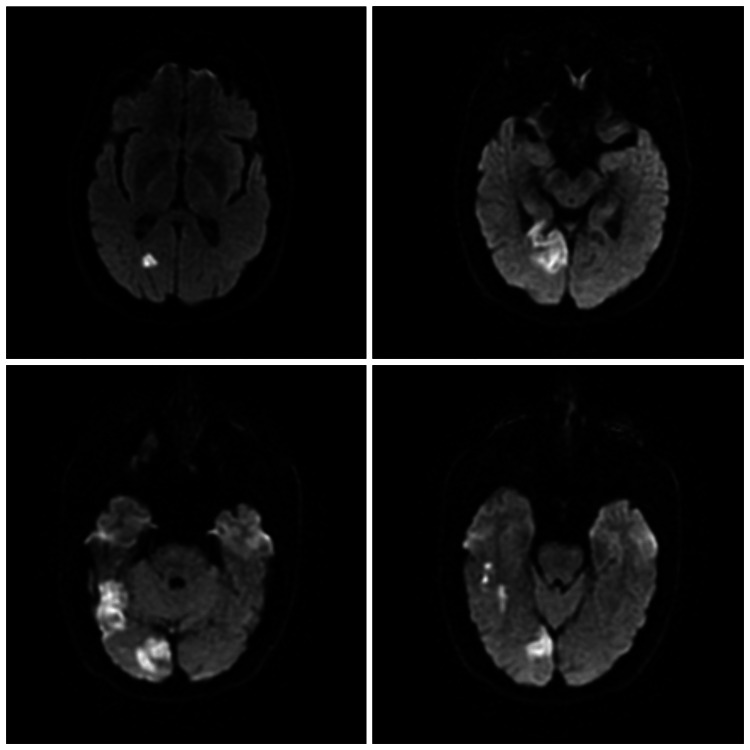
Diffusion-weighted MRI sequence showing increased signal intensity in the right occipital and right posterior temporal region MRI: magnetic resonance imaging
